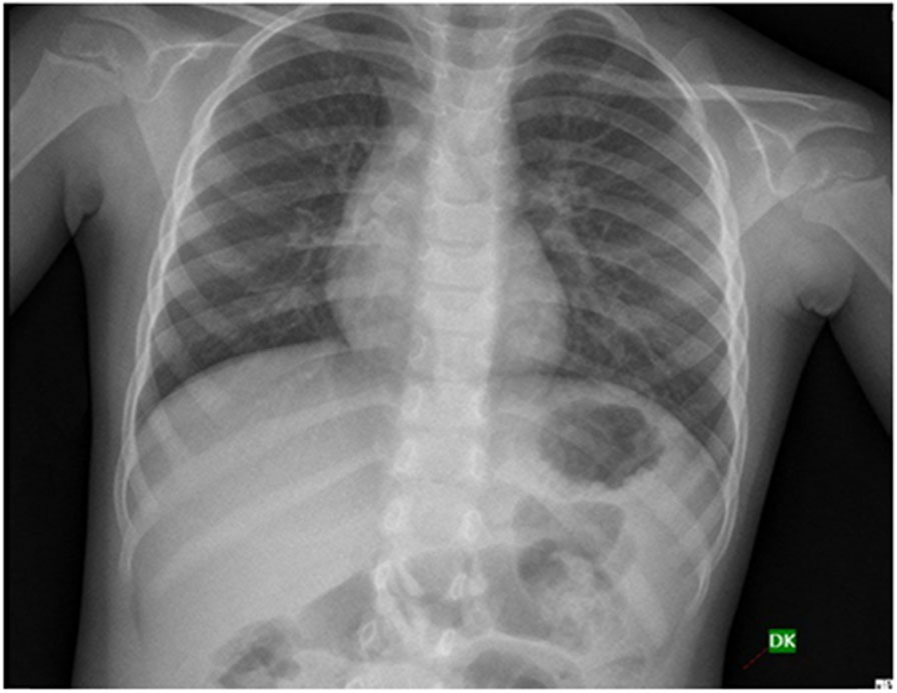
Normal Chest x-ray in a case of early FBA
